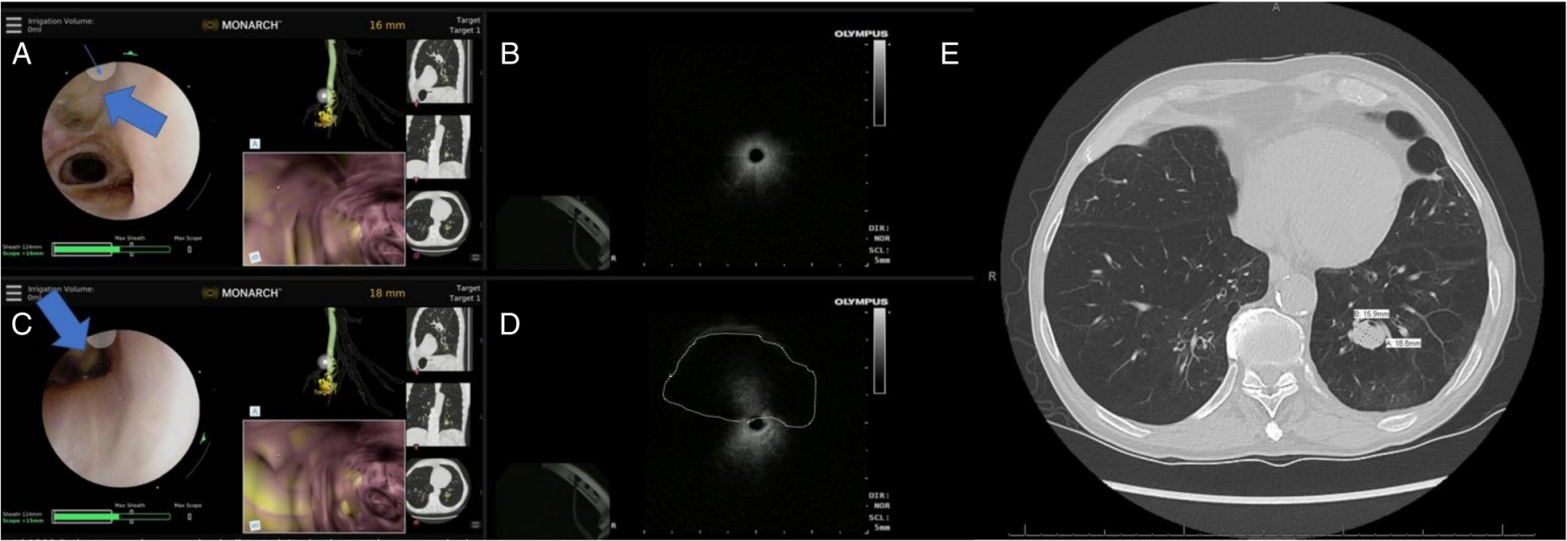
REBUS- nodule relationship during robotic bronchoscopy. a REBUS probe (thick arrow) is advanced under direct visualization and seen exiting the scope at the site of the indicator (at 11 o’clock position on the screen- thin arrow). b in that airway, the REBUS image only showed air artifact. c In the same location, the REBUS probe is then oriented towards 6 O’clock position on the screen. The probe is in partial contact with the airway wall (thick arrow). d In that position, REBUS screen shows the nodule as an isoechoic image- highlighted with a dashed line). It is also obvious that nodule as seen on REBUS, although it appears to be at 12 o’clock position relative to the probe, it is clearly at 6’o’clock position as that is where the probe touches the airway wall. This relationship is crucial to identify as the needle orientation is dependent on it. e The panel shows the chest CT scan with the nodule biopsied in this case (a 15.9 X 18.8 mm nodule in RB10) REBUS: radial probe ultrasound
